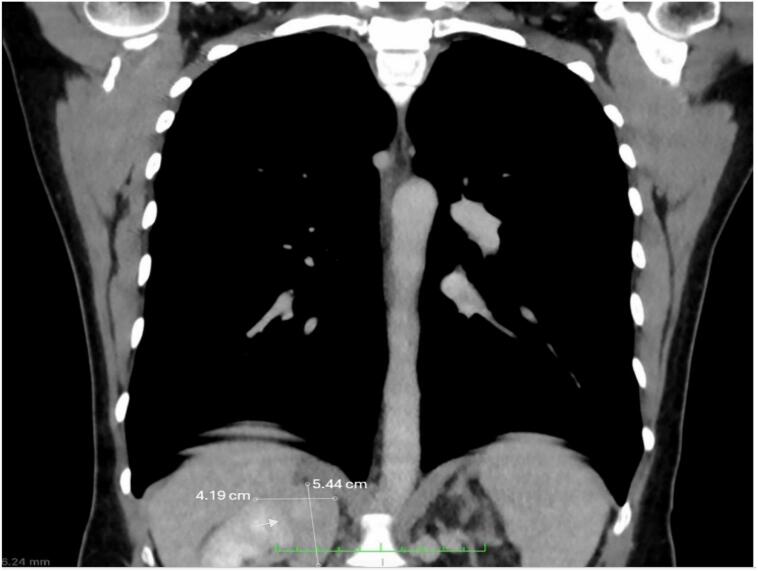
Coronal view of chest CT scan showing an enhancing mass measuring 5.4 × 4.1 cm. The mass is said to project along the right suprarenal aspect or exophytic from the superior right renal cortex.
